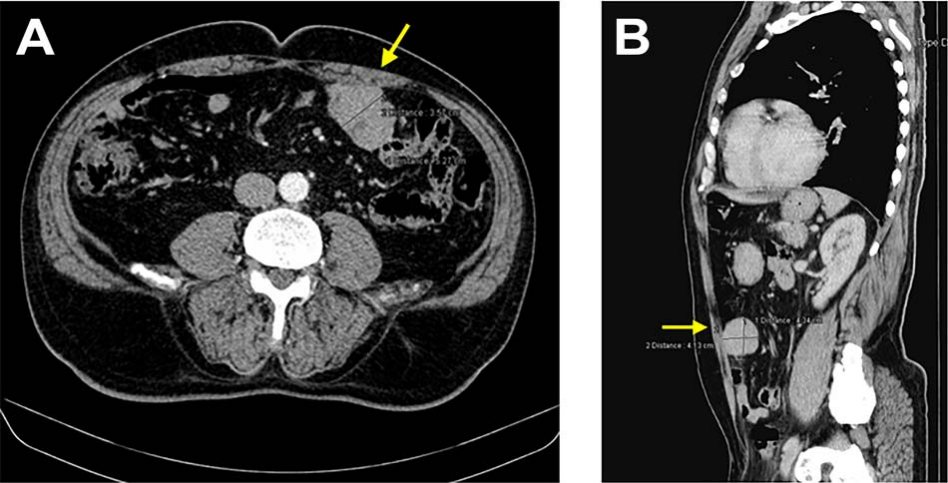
CT images showing a nodular lesion with 48 × 57 × 44 mm, close to the anterior abdominal wall (yellow arrow).
